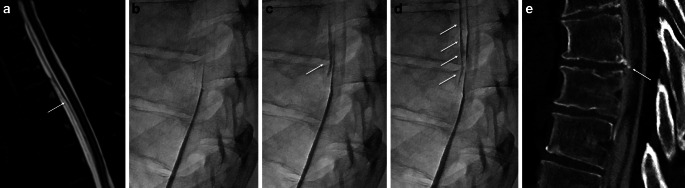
Middle-aged patient with sudden onset of orthostatic headache. a Heavily T2 weighted sagittal MRI with visible cerebrospinal fluid in the ventral epidural space, and the dura mater (*arrow*). b–d Dynamic myelography with contrast medium leaking into the ventral epidural space (*arrow*). e Postmyelography CT demonstrating a ventral microspur (*arrow*) at the corresponding level leading to a dural breach
